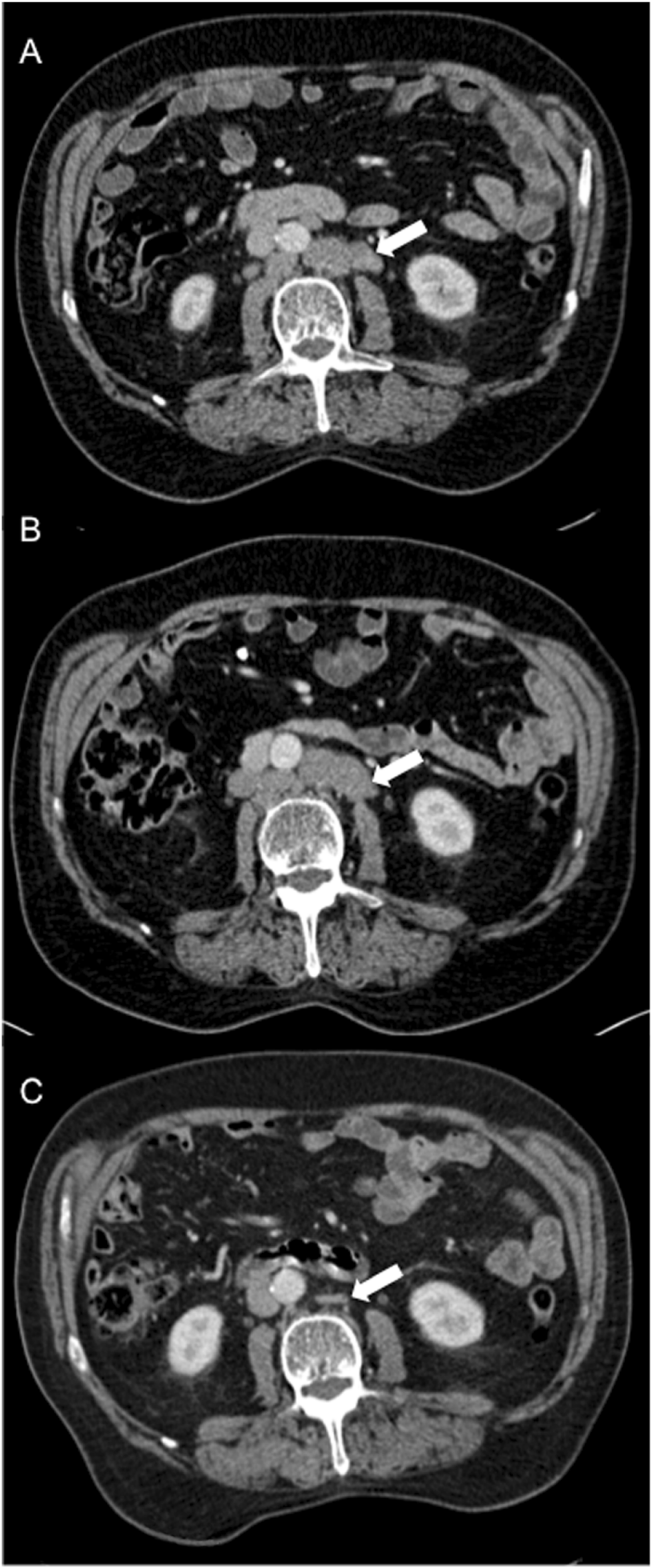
Serial abdominal computed tomography scans. (A) Baseline computed tomography scan performed 3 days prior to the start of chemotherapy. Bulky retroperitoneal lymph nodes are visible (arrow). (B) End-of-treatment computed tomography scan performed 28 days following the last dose of chemotherapy. The lymphadenopathy persists (arrow) and was deemed stable by Response Evaluation Criteria In Solid Tumours criteria. (C) Follow-up computed tomography scan, performed 203 days following the last dose of chemotherapy. This scan was requested in view of the dramatic fall in PSA. Complete resolution of the lymphadenopathy is observed, with only a small residuum left (arrow).
